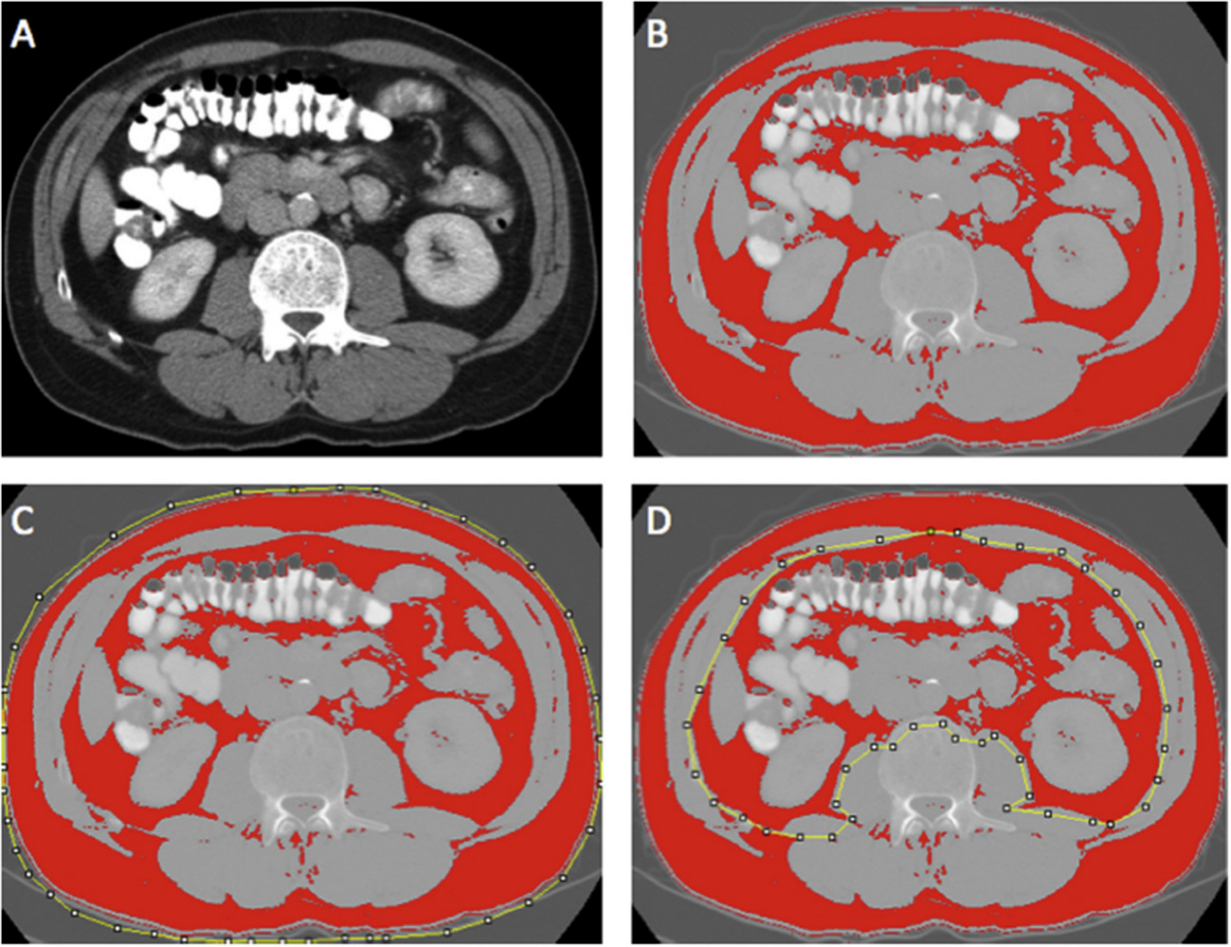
Example of selection of CT body composition—fat areas using ImageJ software; (A) mid-L3 vertebra axial slice from preoperative portal venous phase CT, (B) threshold selection of adipose tissue using automatic selection of pixels of radiodensity ranging −190 to −30 Hounsfield units (HU); (C) region of interest (ROI) selection for total fat area (TFA, cm^2^); and (D) ROI selection for visceral fat area (VFA, cm^2^). Adapted from McSorley et al. (22).
